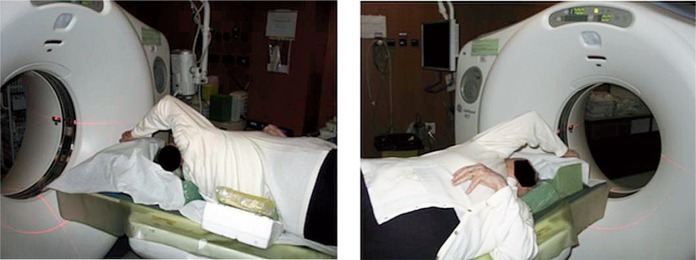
Position of the patient in the CT scanner as used in this study: patient in lateral decubitus or in three-quarter decubitus, allowing the scapula to tilt, with shoulder in maximal flexion.
